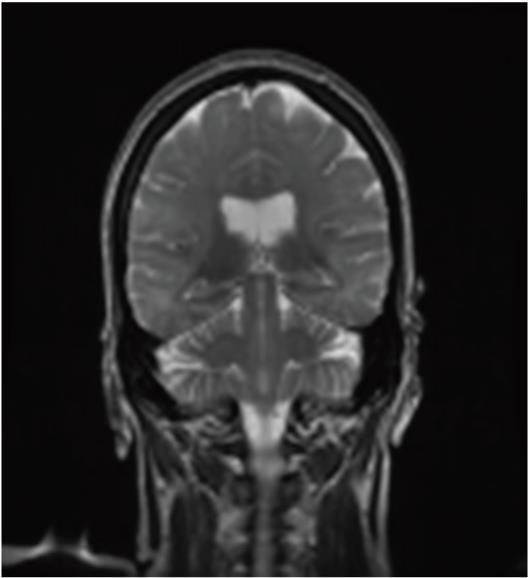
Coronal FSE T2 image on repeat MRI revealing extensive subcortical white matter hyperintensity in the midline parietal cortex, cerebellum, and brainstem.
